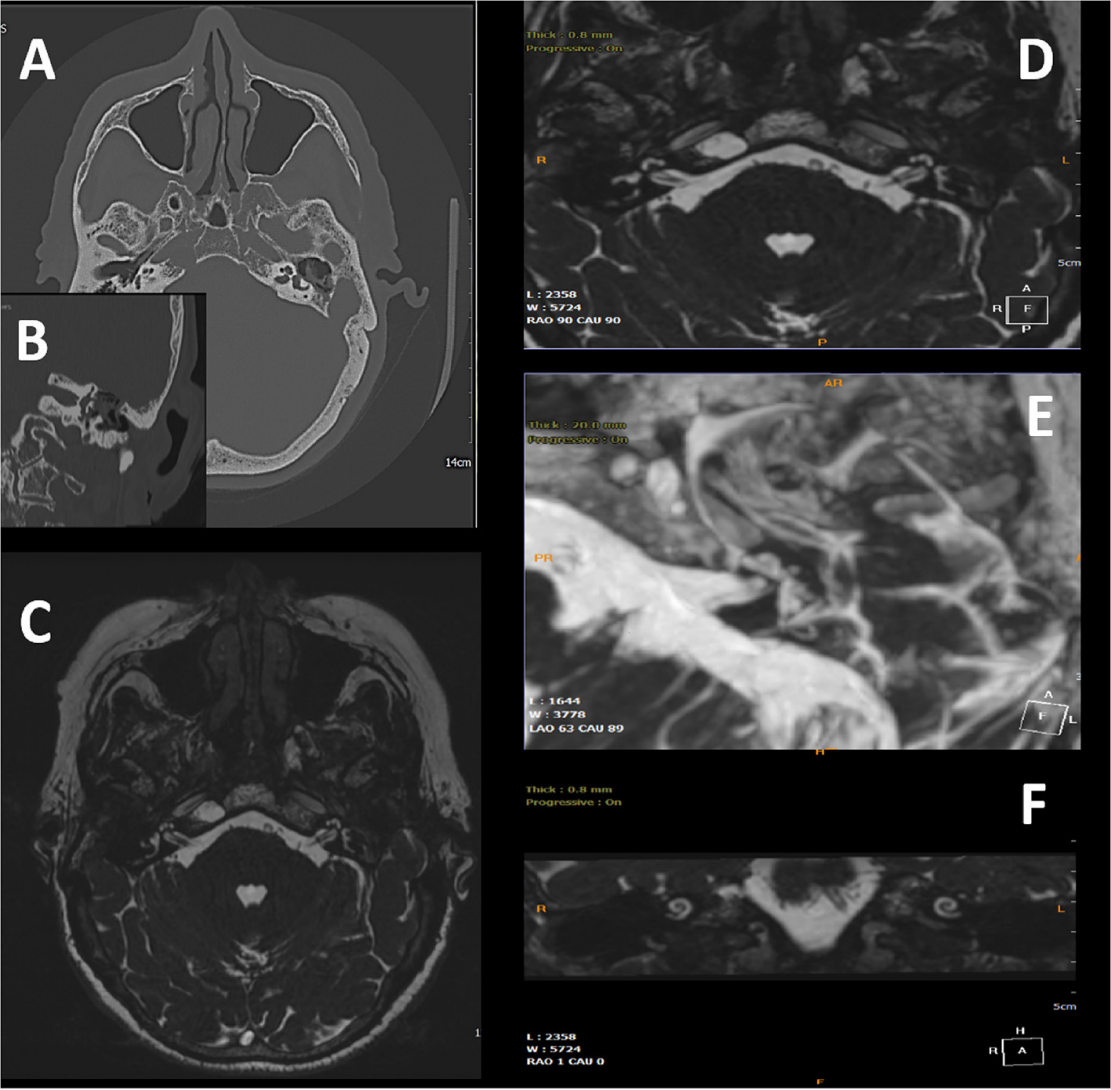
75-year-old woman; (A) pre-operative axial CT scan showing the cholesteatomatous tissue and erosion of semicircular canals. (B) Particular coronal CT scan showing the erosion of the lateral semicircular canal, and the erosion of the tympanic tegmen; (C,D) post-operative images with MRI 3D Fiesta 3D sequences (field of view 20 × 20 cm TR 4 ms; TE 2 ms; flip angle 55°); (E) MIP for cochlea and semicircular canal visualization, detail of the operated side shows the correct visualization of the preservation of endocanal fluids. (F) MRI Coronal image with preservation of endocanal fluids, meaning preservation of hearing.
